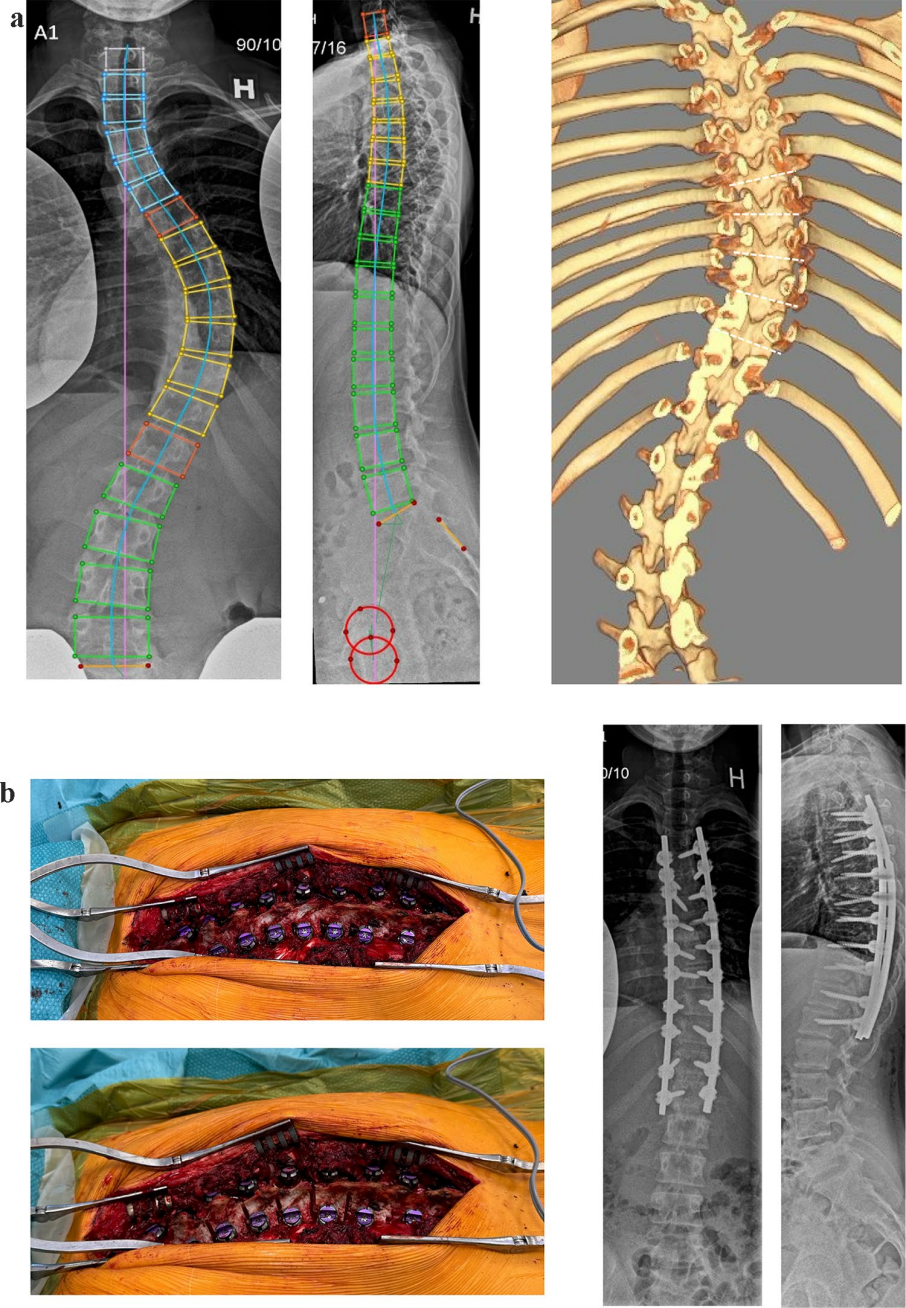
a Preoperative X-ray of a Lenke type 1A curve with a substantial hypokyphosis. On the right is the 3-D reconstructive CT suggesting the site and trajectory of the posterior release corresponding to the apex of the curve (dotted lines). b Intraoperative image of the thoracic spine before (left) and after (right) posterior release. Postoperative X-ray showing normalization of thoracic kyphosis
